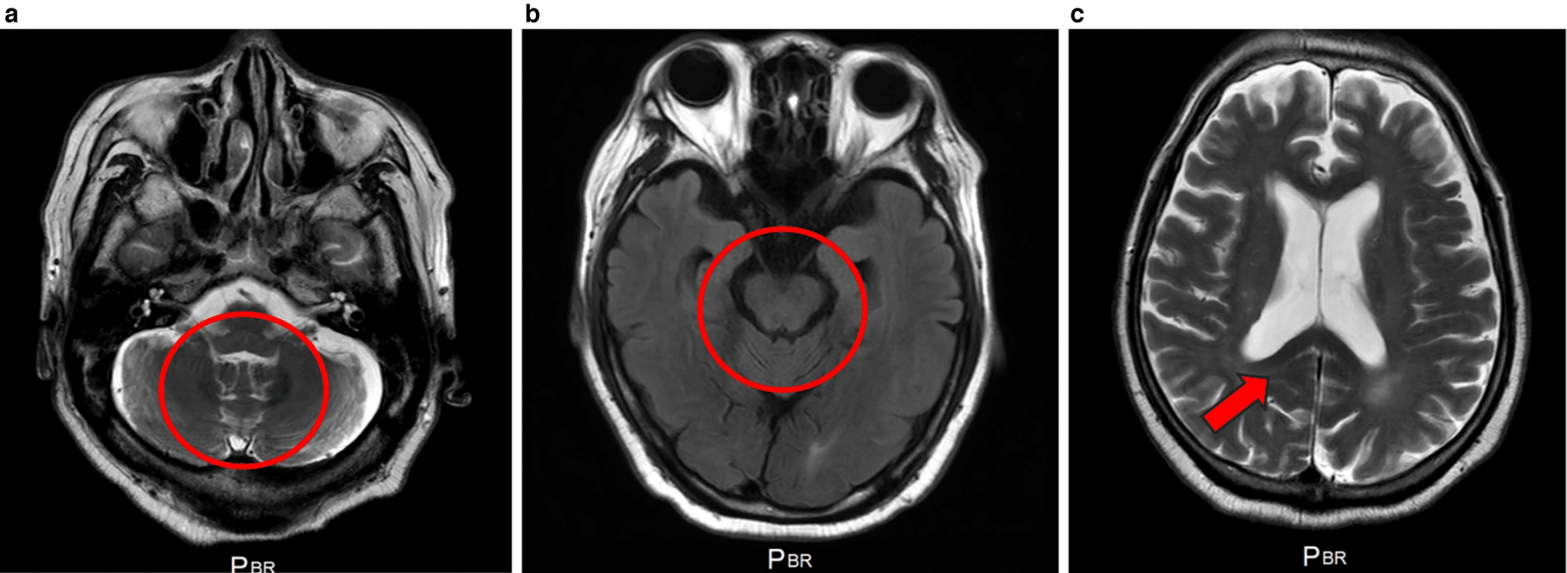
Follow-up brain MRI on day 92 after the discontinuation of metronidazole Axial FLAIR images (a, b; red circles) and an axial T2-weighted image (c; red arrow) demonstrate complete resolution of the previously observed symmetric hyperintensities in the cerebellar dentate nuclei and dorsal midbrain tegmentum, with the disappearance of the faint splenial lesion in the corpus callosum. The radiologic reversibility parallels the patient's clinical recovery and is characteristic of metronidazole-induced encephalopathy. FLAIR: fluid-attenuated inversion recovery; MRI: magnetic resonance imaging
